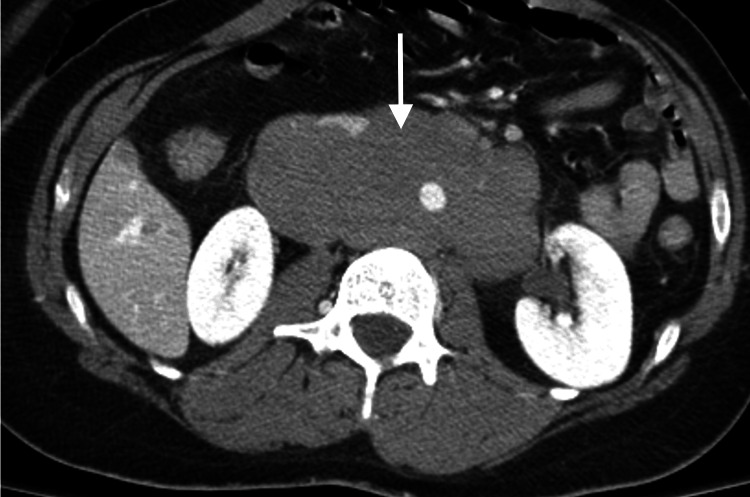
CT scan of the abdomen with contrast demonstrating extensive DVTs in the left hemipelvis (white arrow) CT: computed tomography; DVT: deep vein thrombosis
